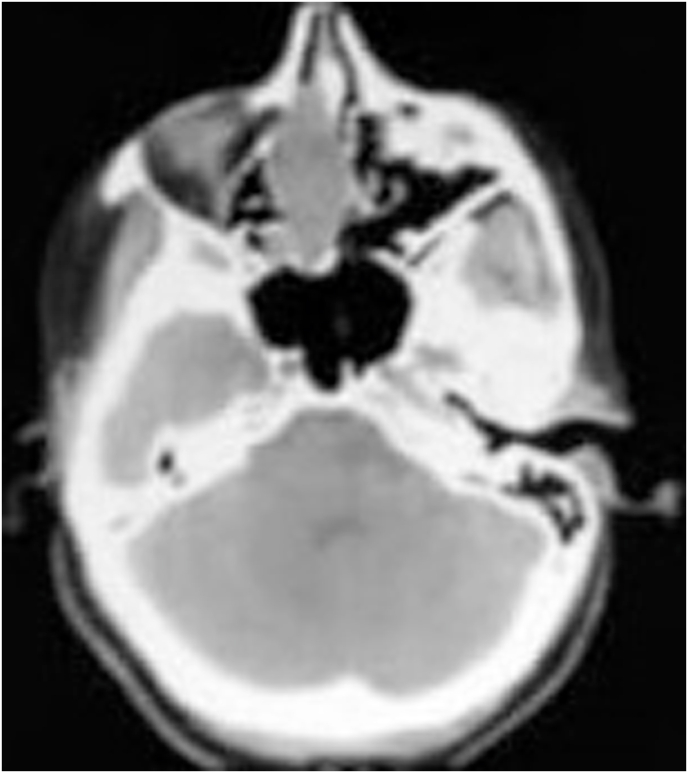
(CT) scan demonstrated an expansive process occupying the ethmoidal cells.
